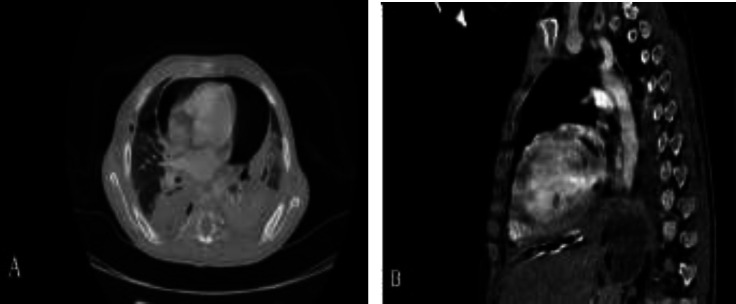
Contrast enhanced chest computed tomography (CT) scan of patient after pericardial catheter and right sided chest tube insertion. Pneumopericardium, right sided pneumothorax as well as bilateral mild pleural effusion and collapse consolidation are evident. A: Axial view. B: Parasagittal view
